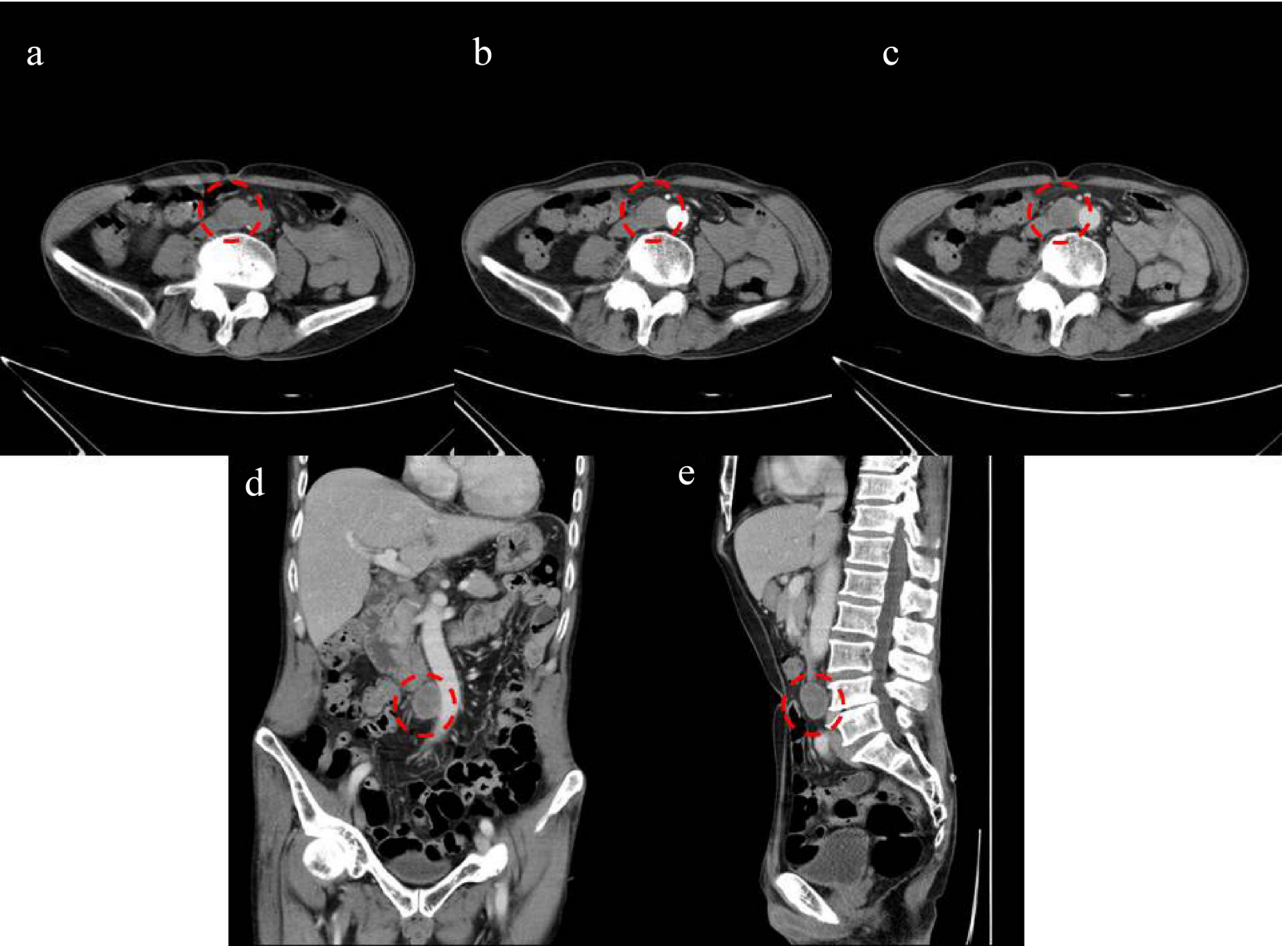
Contrast-enhanced CT of the abdomen. (a) Noncontrast-enhanced CT scan revealed an isointense solid mass with a clear boundary adjacent to the abdominal aorta (red dashed circle); (b and c) arterial and venous phase enhancement scans with mild enhancement of the mass (red dashed circle); (d and e) multiplanar reconstructed sagittal and coronal views revealed the adjacent relationship between the mass and the surrounding structures (red dashed circle).
